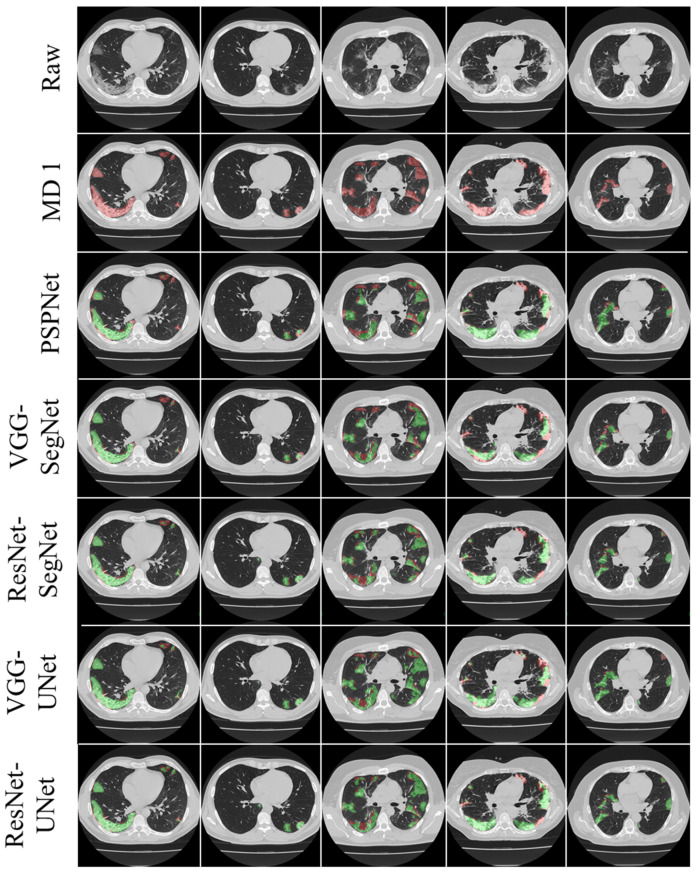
Row 1: raw CT; row 2: MD 1 (gold standard); rows 3–7: overlay images—AI (green) over MD (red). The 5 AI models are PSPNet (row 3), VGG-SegNet (row 4), ResNet-SegNet (row 5), VGG-UNet (row 6), and ResNet-UNet (row 7).
